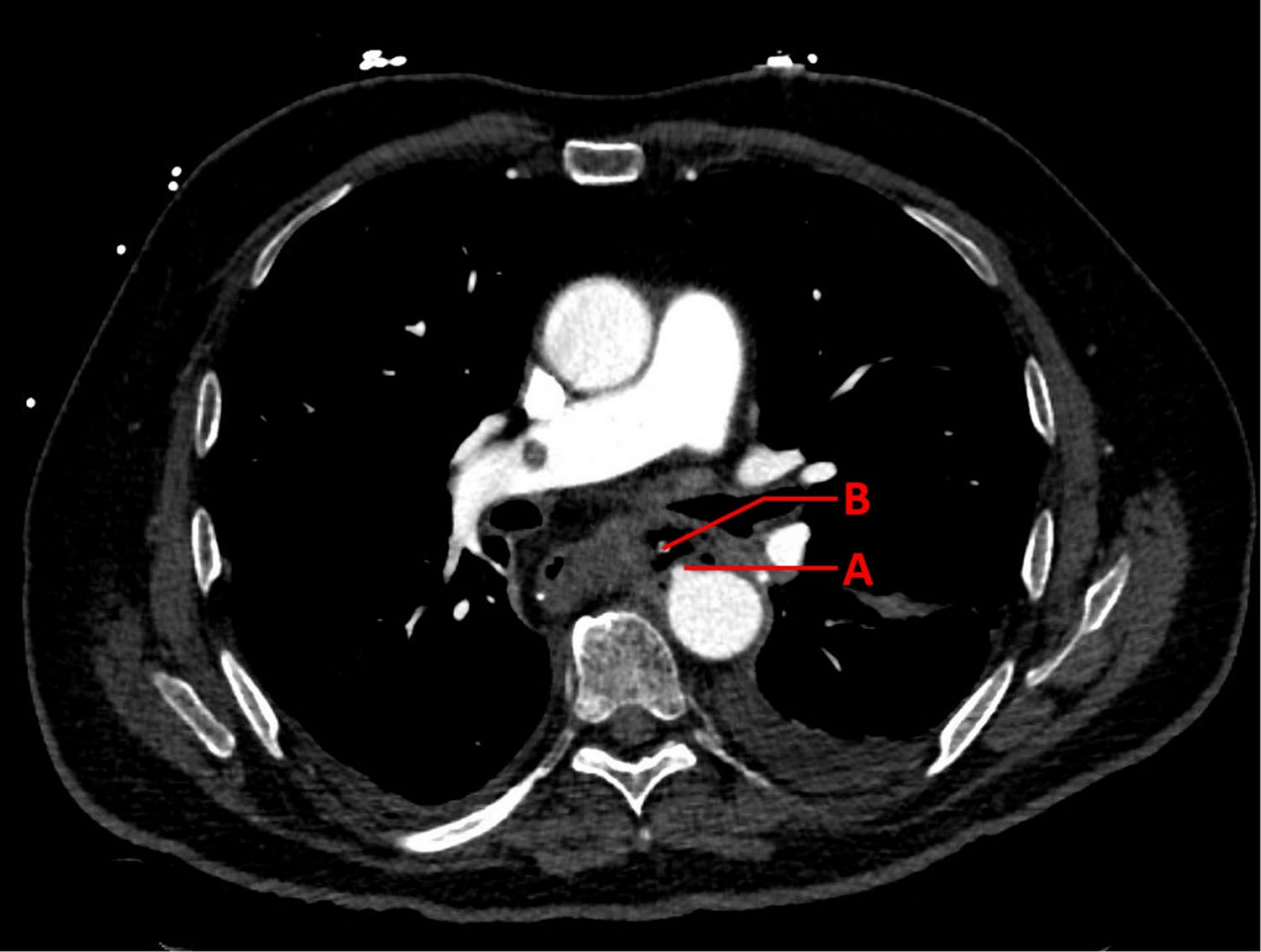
CT angiography with aortic blush (A) on the ascending aorta adjacent to the intrathoracic anastomosis with extraluminal vacuum-sponge in the mediastinum (B)
